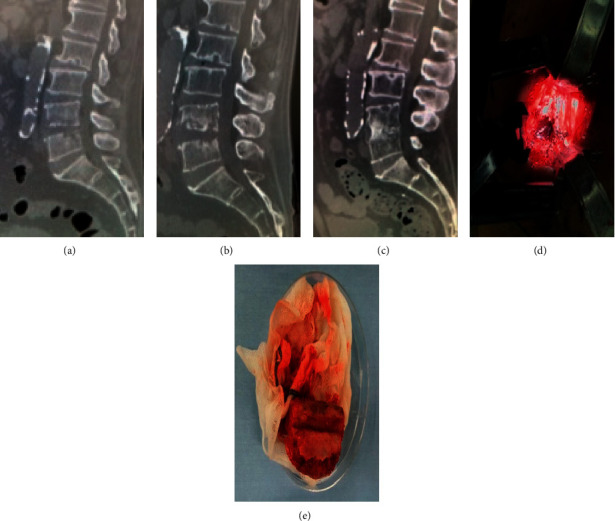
A 75-year-old male with L4/L5 pyogenic spondylitis underwent endoscopic debridement. Because low back pain did not improve postoperatively, anterior reconstruction surgery with an iliac autograft was performed. (a) Preoperative sagittal CT scans revealed destructive endplate at the L4/L5 level. (b) Post-endoscopic surgery sagittal CT scans showing further bone destruction. (c) Post-open surgery sagittal CT scan view showing the bony fusion without severe kyphotic deformity.(d, e) Intraoperative view and iliac autograft; minimally invasive surgery was performed via the direct lateral retroperitoneal approach, and the large defect due to debridement was reconstructed with the strut bone collected from the ilium.
